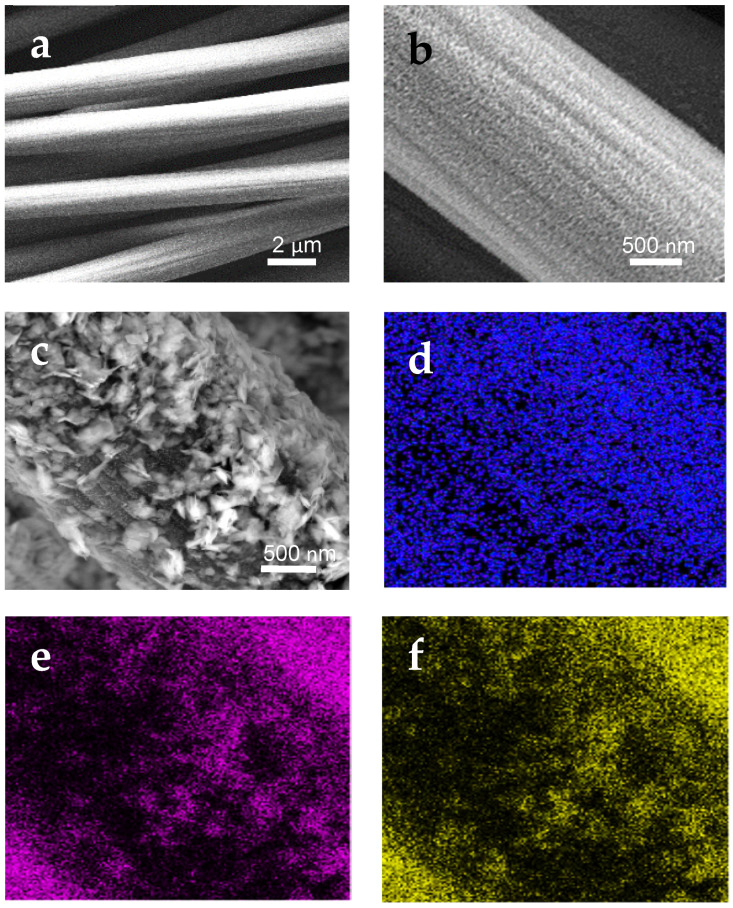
SEM images of: (a) CC/SPCE; (b) MoO_3_-CC/SPCE; (c) MoS_2_-MoO_3_-CC/SPCE. (d–f) energy dispersive X-ray (EDX) mapping of the SEM image shown in (c) at the O, Mo, and S energies, respectively.
